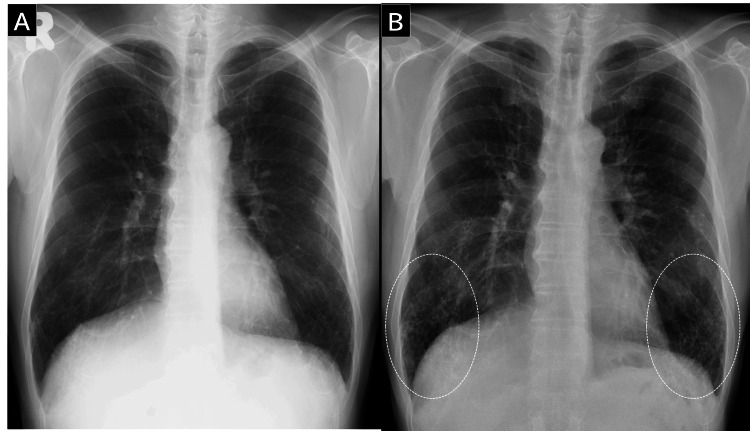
Chest radiography findings over time (A) Chest radiograph at medical check-up. (B) The bilateral reticulonodular shadows in the lower lung zone (circle) became clearer after five years.
